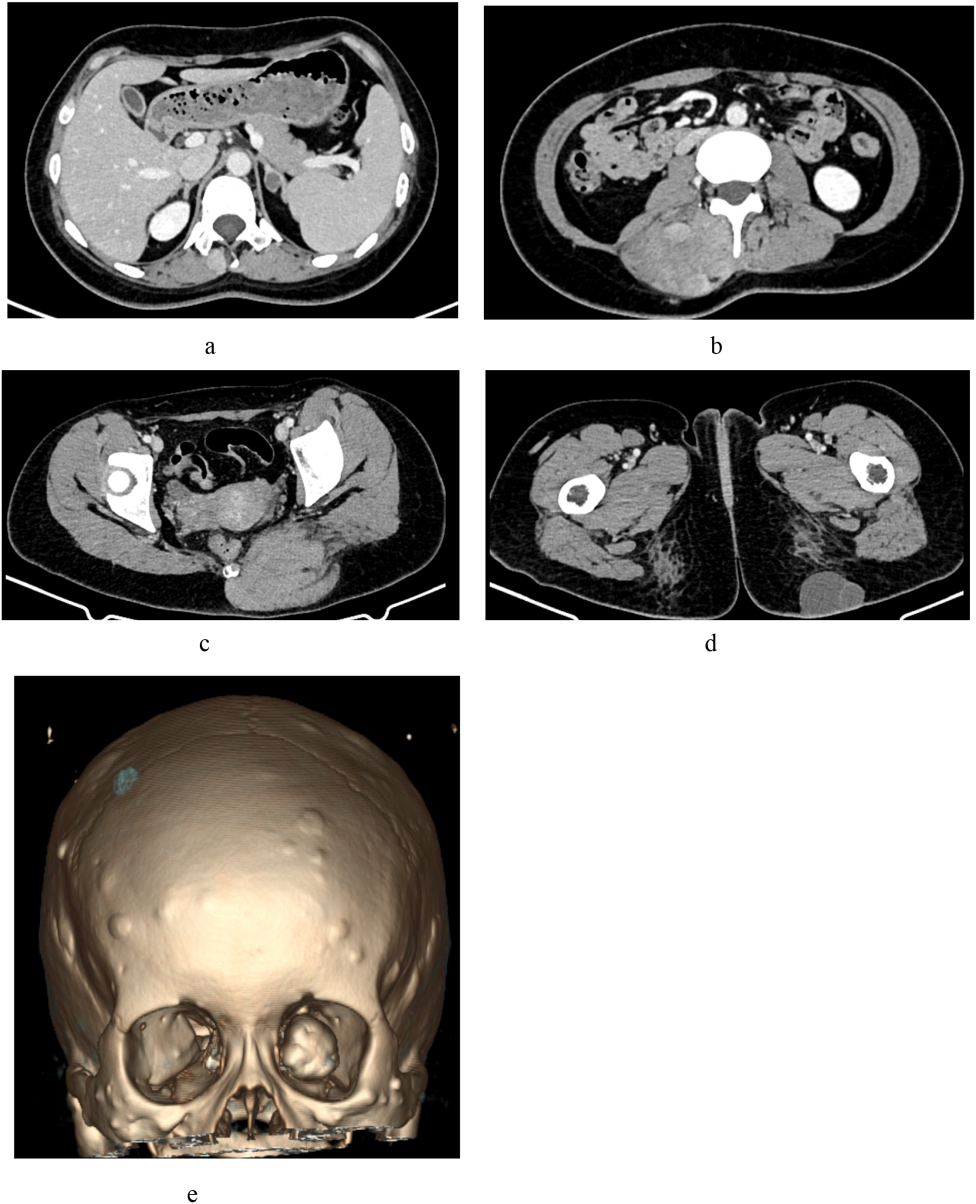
Contrast-enhanced computed tomography findings. (a) Left adrenal adenoma: A small, well-defined nodule in the left adrenal gland (maximum diameter: 14 mm). (b) Right erector spinae soft tissue mass: A heterogeneously enhancing lesion with ill-defined muscular boundaries. (c) Left gluteus maximus soft tissue mass: An irregular, heterogeneously enhancing intramuscular lesion. (d) Left gluteal subcutaneous cyst: A non-enhancing, hypodense cystic lesion within the subcutaneous tissue. (e) Craniofacial osteomas: Multiple osteogenic lesions involving the left orbital region and cranial bones.
